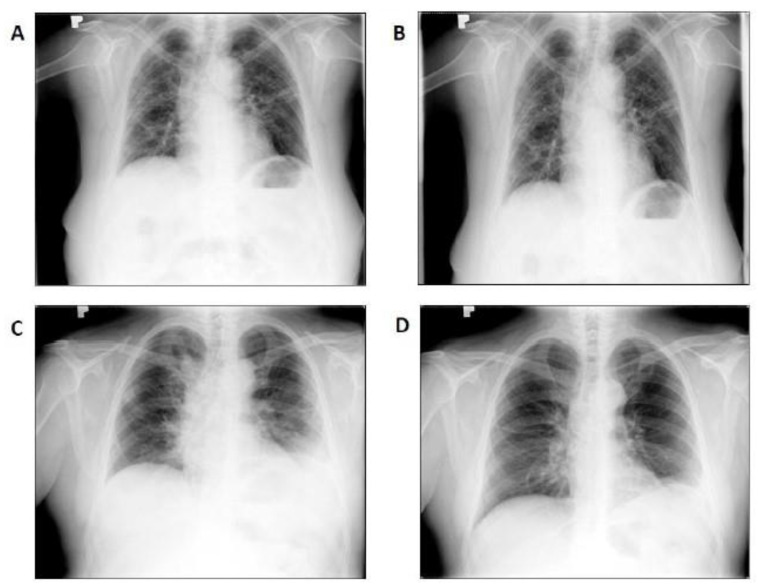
Chest radiographs of first two consecutive patients with COVID-19-associated pneumonia included in the study prior to and 14 days after LDRT procedure showing radiological improvement. ((A)—patient #1 baseline, (B)—patient #1 day 14, (C)—patient #2 baseline, (D)—patients #2 day 14).
